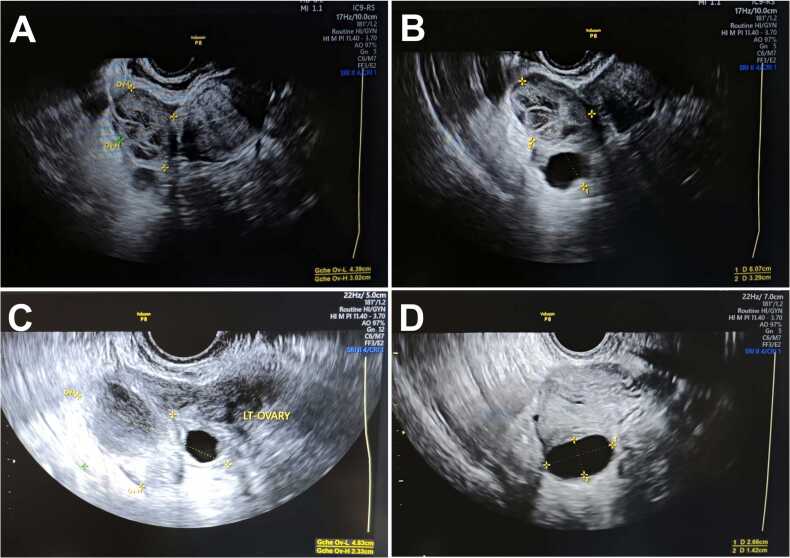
Transvaginal ultrasound. (A) Longitudinal B-mode view showing a multiloculated cystic mass (48 × 32 × 30 mm) in the left ovary. (B) Transverse view (zoom) with thin septations and multiple locules without vegetations. (C) Additional view confirming multiloculation with smooth walls. (D) Detail of an isolated locule with thin wall and homogeneous anechoic content.
